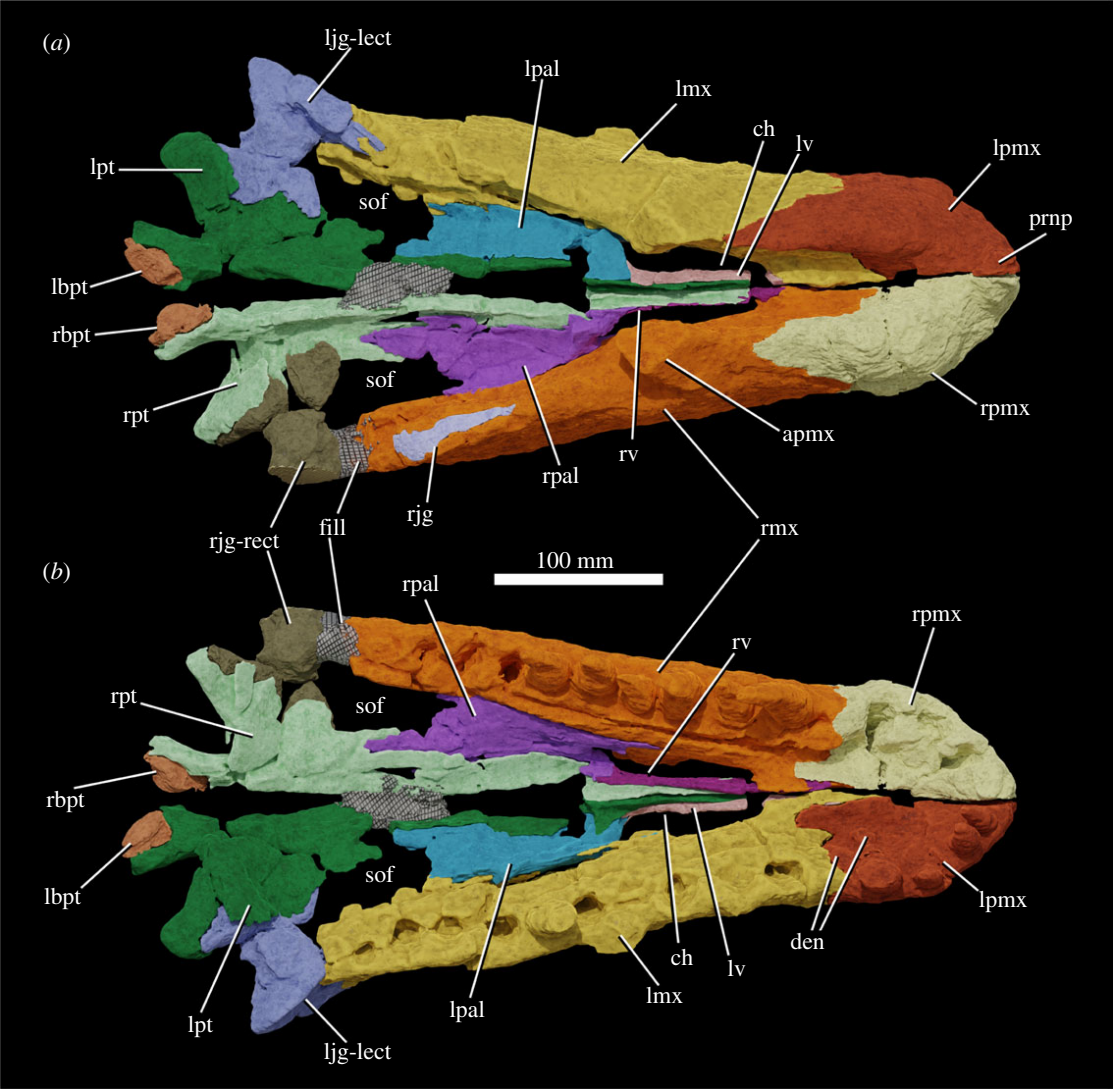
Segmentation based on CT data of the skull of NHMUK R36620, holotype of *Mambawakale ruhuhu*, in dorsal (*a*) and ventral (*b*) views. Abbreviations: apmx, ascending process of the maxilla; ch, choana; den, dentary tooth fragments attached to the premaxilla; fill, area of artificial repair; lbpt, left basipterygoid process of the basisphenoid; ljg-lect, left jugal and left ectopterygoid; lmx, left maxilla; lpal, left palatine; lpmx, left premaxilla; lpt, left pterygoid; lv, left vomer; prnp, prenarial process of the premaxilla; rbpt, right basipterygoid process of the basisphenoid; rjg, fragment of the right jugal; rjg-rect, right jugal and right ectopterygoid; rmx, right maxilla; rpal, right palatine; rpmx, right premaxilla; rpt, right pterygoid; rv, right vomer; sof, suborbital fenestra.
